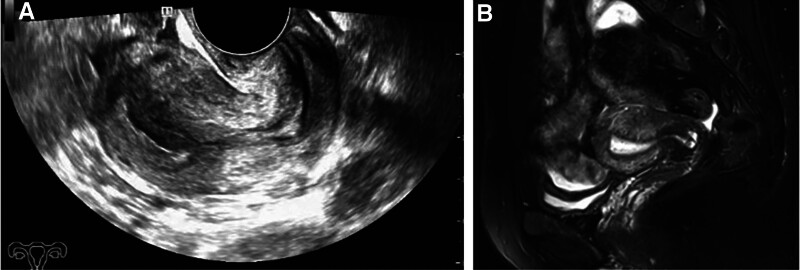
Pelvic TVS (A) and MRI (B) show that the uterus contained normal endometrium and myometrium 3 months after the operation (case 1). MRI = magnetic resonance imaging, TVS = transvaginal ultrasonography.
